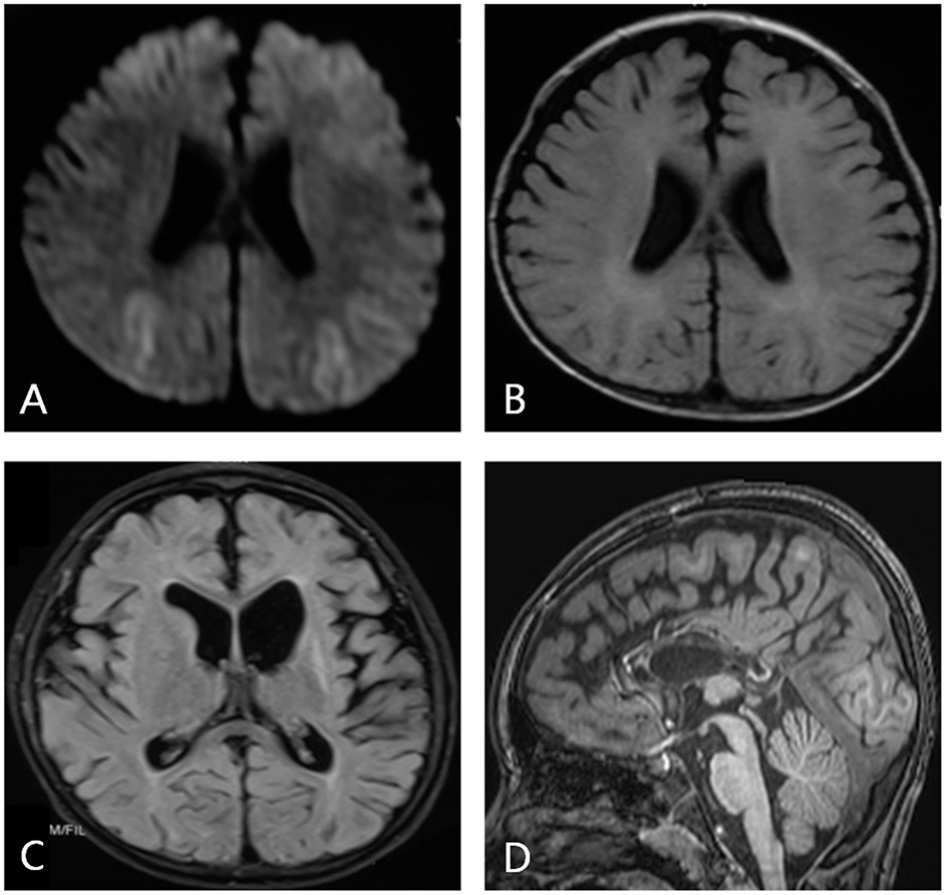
Neuroimaging data of case 2. During the acute phase of viral encephalitis, MRI shows an abnormal signal in the bilateral frontal and parietal regions on diffusion-weighted imaging (A) and T1-weighted imaging (B). MRI reveals a more obvious signal on the left side, and dilation of the left lateral ventricle is observed (C). Imaging shows completed corpus callosotomy (D).
